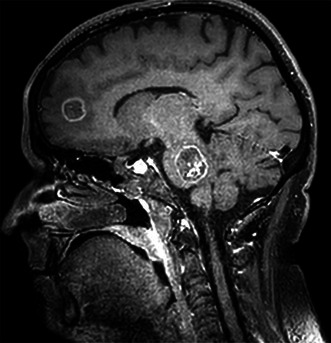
FIGURE 2 MRI Brain ‐ T2 FLAIR sagittal section showing ring enhancing lesion with intracavitary projections in frontal lobe and brainstem.
